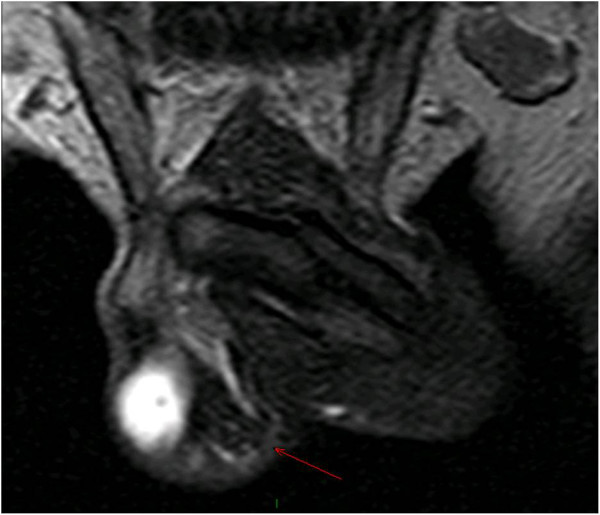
Coronal T2-weighted TSA MR imaging showing fibrotic reaction (red arrow) extended in the connective tissue of the scrotal sac.
